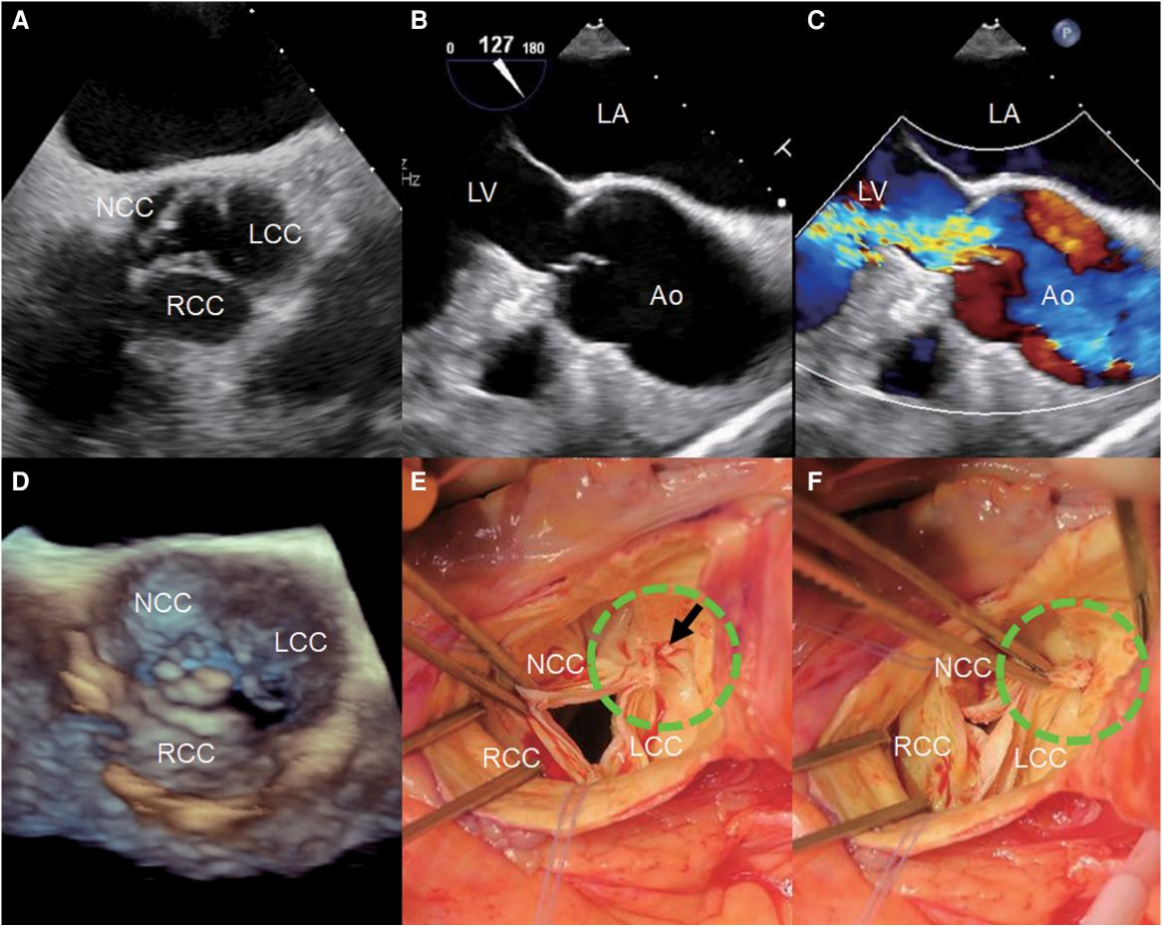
Transoesophageal echocardiography and intraoperative findings. (*A*) Transoesophageal echocardiography short-axis view of the aortic valve. (*B*) Transoesophageal echocardiography long-axis view showing aortic valve prolapse into the left ventricle. (*C*) Colour Doppler transoesophageal echocardiography long-axis view demonstrating severe AR with a broad jet. (*D*) 3D-en-face transoesophageal echocardiography view clearly showing prolapse of the left coronary cusp and noncoronary cusp. Intraoperative images from the aortic side revealed a tear (arrow) in the intima at the commissure between the left coronary cusp and noncoronary cusp (*E*), confirming commissural detachment (*F*). NCC, noncoronary cusp; RCC, right coronary cusp; LCC, left coronary cusp; LV, left ventricle; LA, left atrium; Ao, aorta; TEE, transoesophageal echocardiography.
